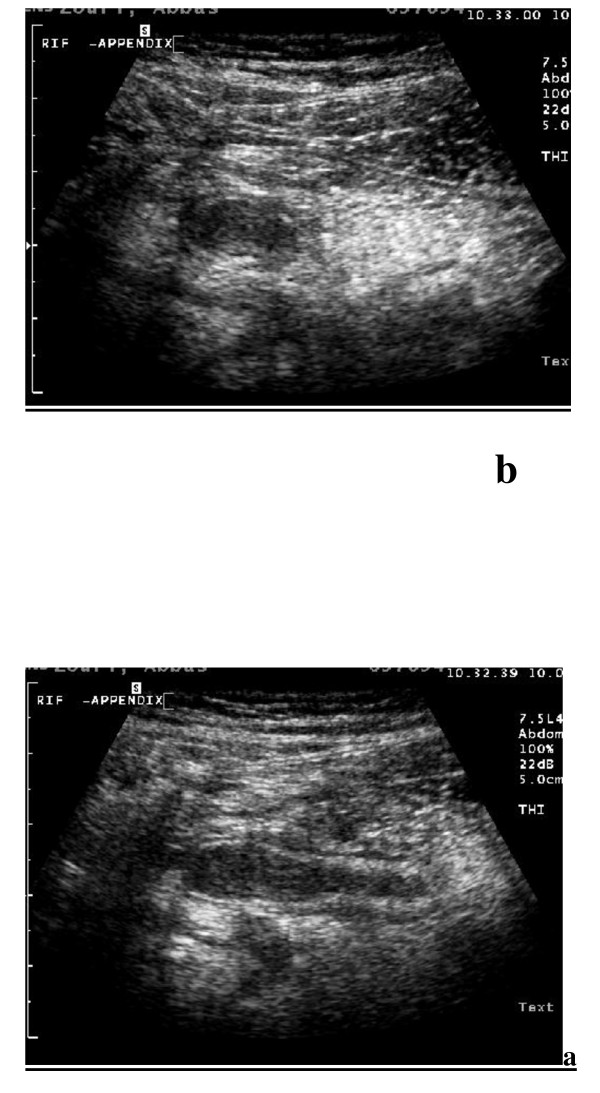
Acute appendicitis by tissue harmonic imaging sonography (THI) using linear transducer with 7.5 MHz revealed: A. Longitudinal scan showing aperistaltic non compressible blind ended tubular structure with distinct thickened wall layers and diameter > 6 mm. B. Transverse scan showing target sign appearance.
